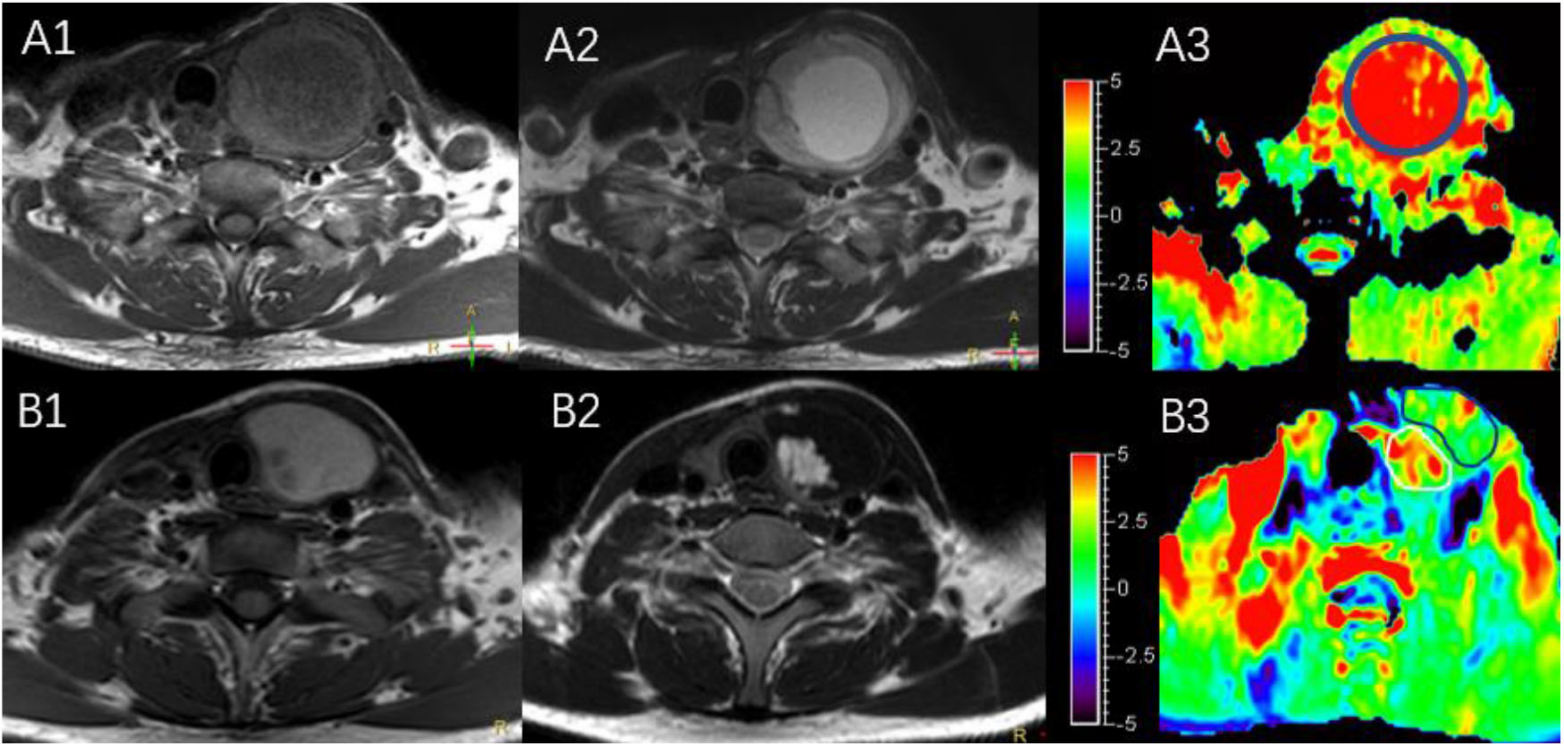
MR images of two predominantly cystic thyroid nodules, including T1WI (A1,B1), T2WI (A2,B2), and APTWI (A3,B3).
